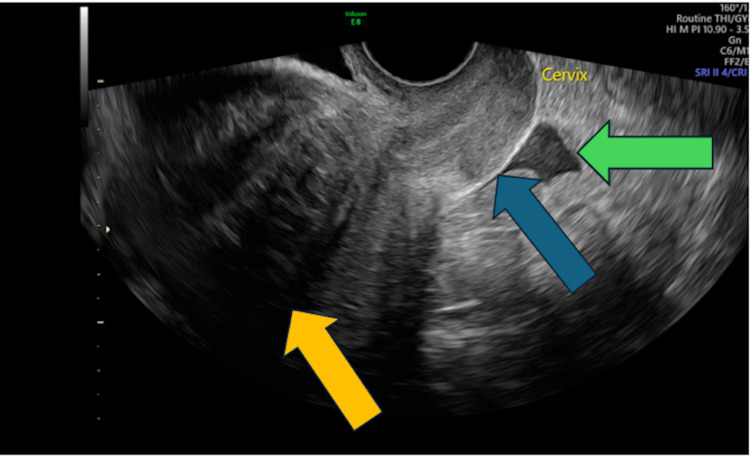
Transvaginal ultrasound: the cervix is seen (blue arrow), with a mild amount of free fluid (green arrow) and a fibroid with shadowing (orange arrow).
